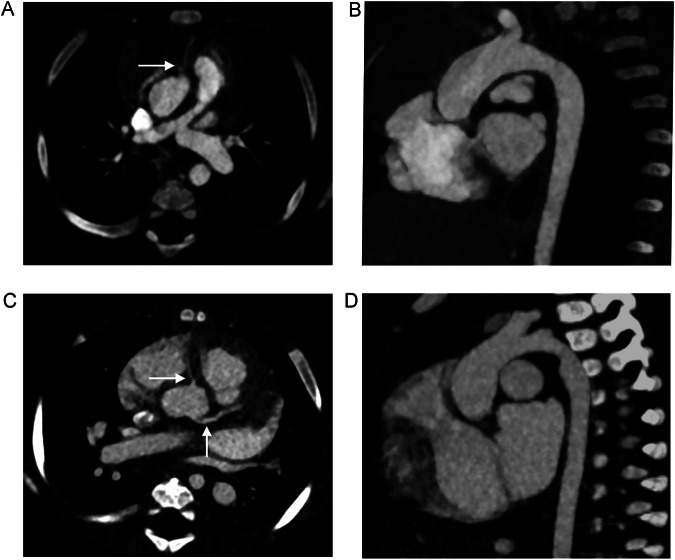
A, B Cardiac examination on photon-counting detector CT of a 7-month-old female patient, born with tetralogy of Fallot. Volume CT dose index (CTDI_vol_) of this examination was 0.11 mGy, dose length product (DLP) was 1.9 mGy*cm, water-equivalent diameter was 118 mm. C, D Cardiac examination on energy-integrating detector CT of a 5-month-old female patient, born with atrioventricular septal defect. CTDI_vol_ of this examination was 0.35 mGy, DLP was 5.8 mGy*cm, water-equivalent diameter was 118 mm. The cardiac anatomy can be very well depicted in both studies, including the coronary arteries (arrows in the axial orientations A and C). B and D show the aortic arch in parasagittal orientation
